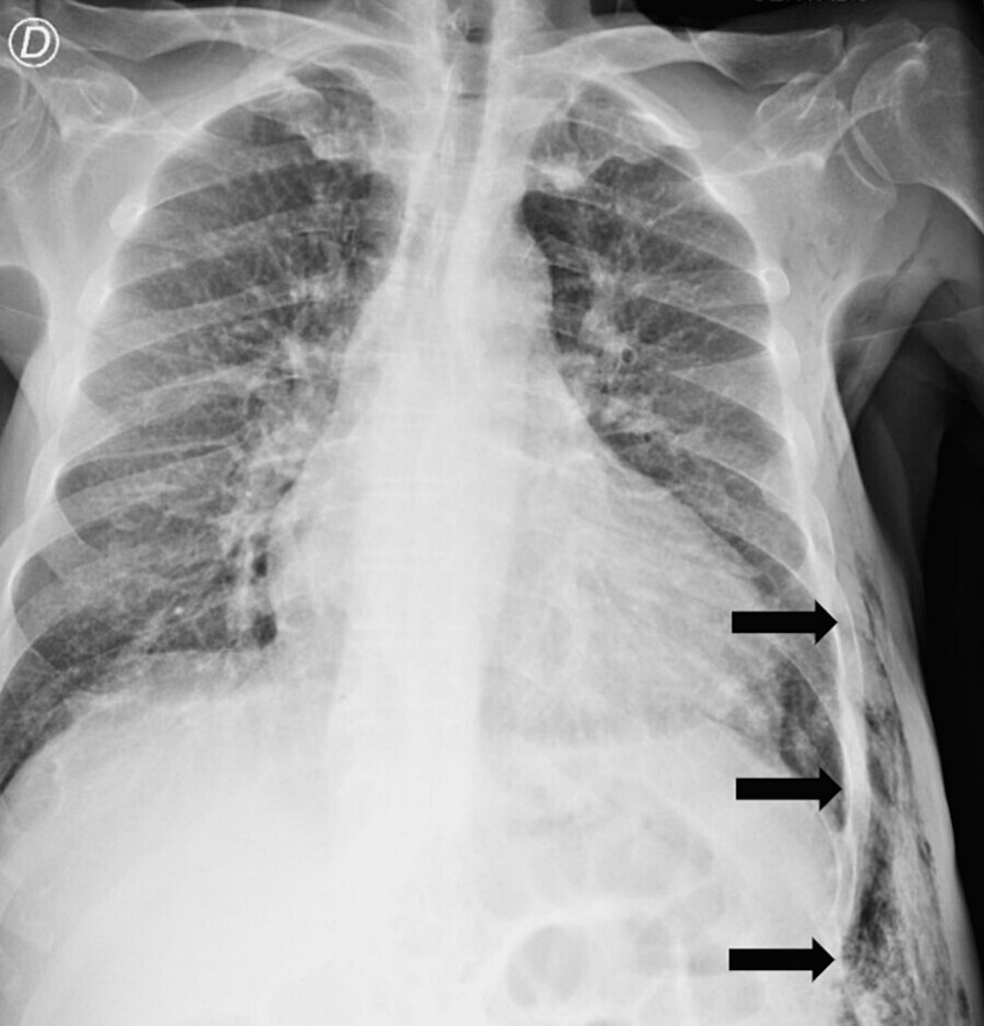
Thoracic radiography: arrows highlighting subcutaneous emphysema in the left side of the thorax and abdomen
